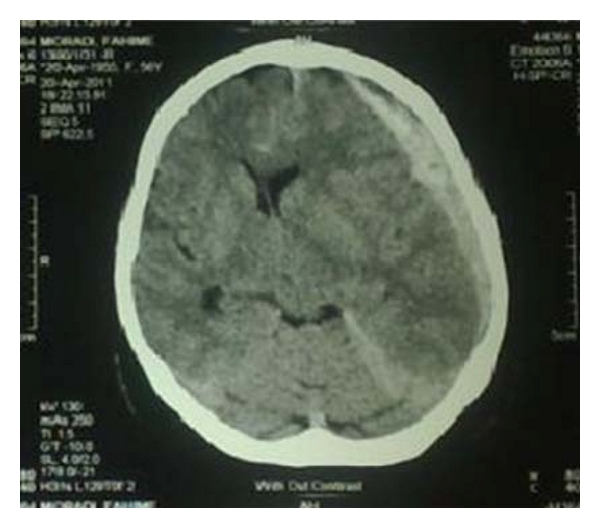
Brain CT scan showed acute SDH of the left frontotemporal region and midline shift.
